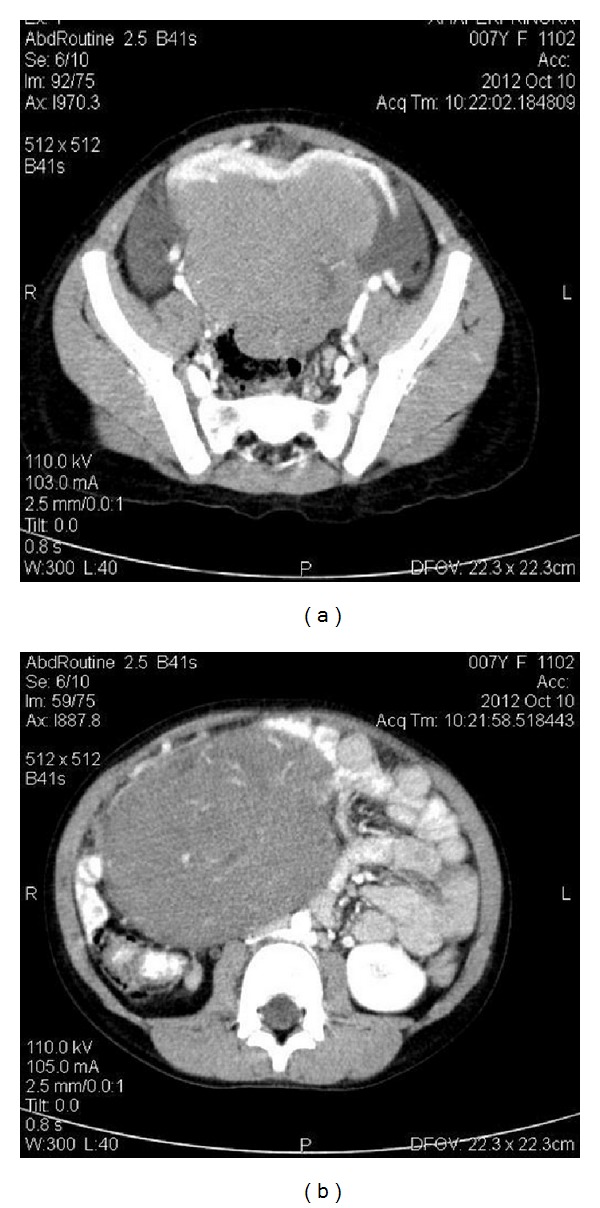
CT scan of the abdomen and pelvis revealed a massive, solid, and clearly bordered formation with the dimensions 12 × 8 × 15 cm. The suspicious formation showed a connection to the right ovary that was hyperdense and had an inhomogeneous appearance. Some hypodense tissue within the tumour mass was identified as probably “fatty tissue.”
